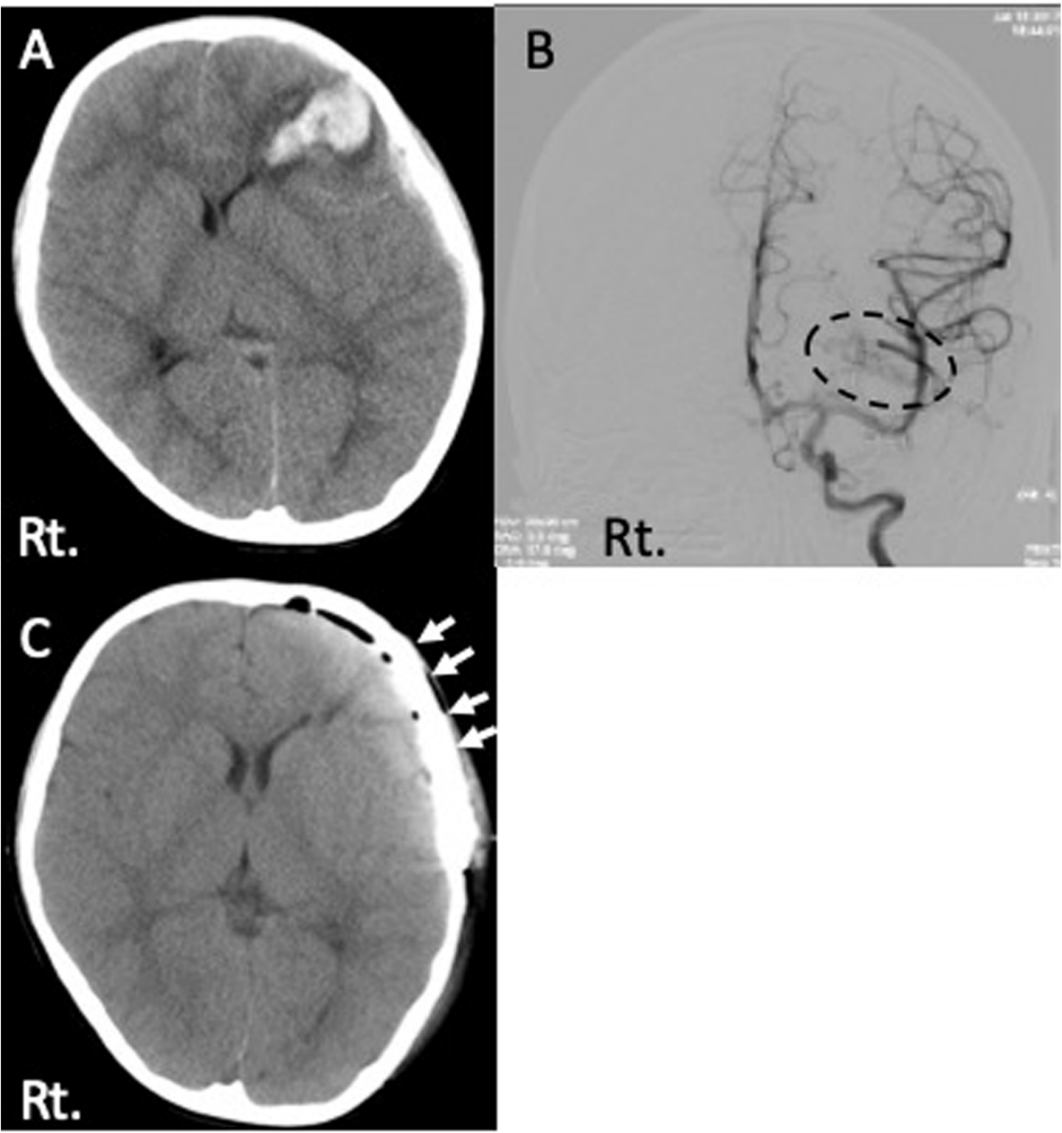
Radiological examinations at first treatment. a Head CT showed left frontal subcortical hematoma. b Cerebral angiography of the left internal carotid artery showed AVM adjacent to the hematoma (dotted ring). c CT image after cranioplasty
